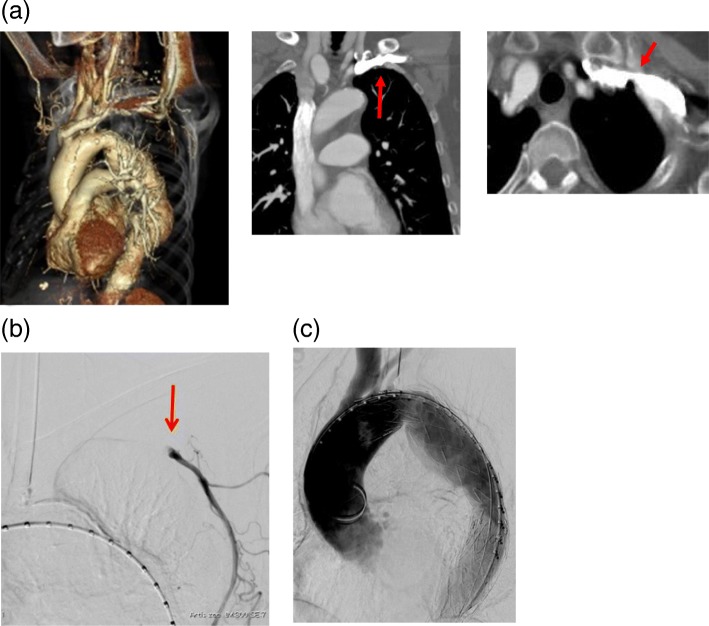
a Preoperative enhanced CT scan failed to visualize AAO. (Left innominate vein’s enhancement concealed the presence of AAO.) Contrast was injected from the left upper extremity, and this caused severe halation. b The AAO was encountered during LSCA angiography (arrow). c There were no endoleaks on completion angiography
